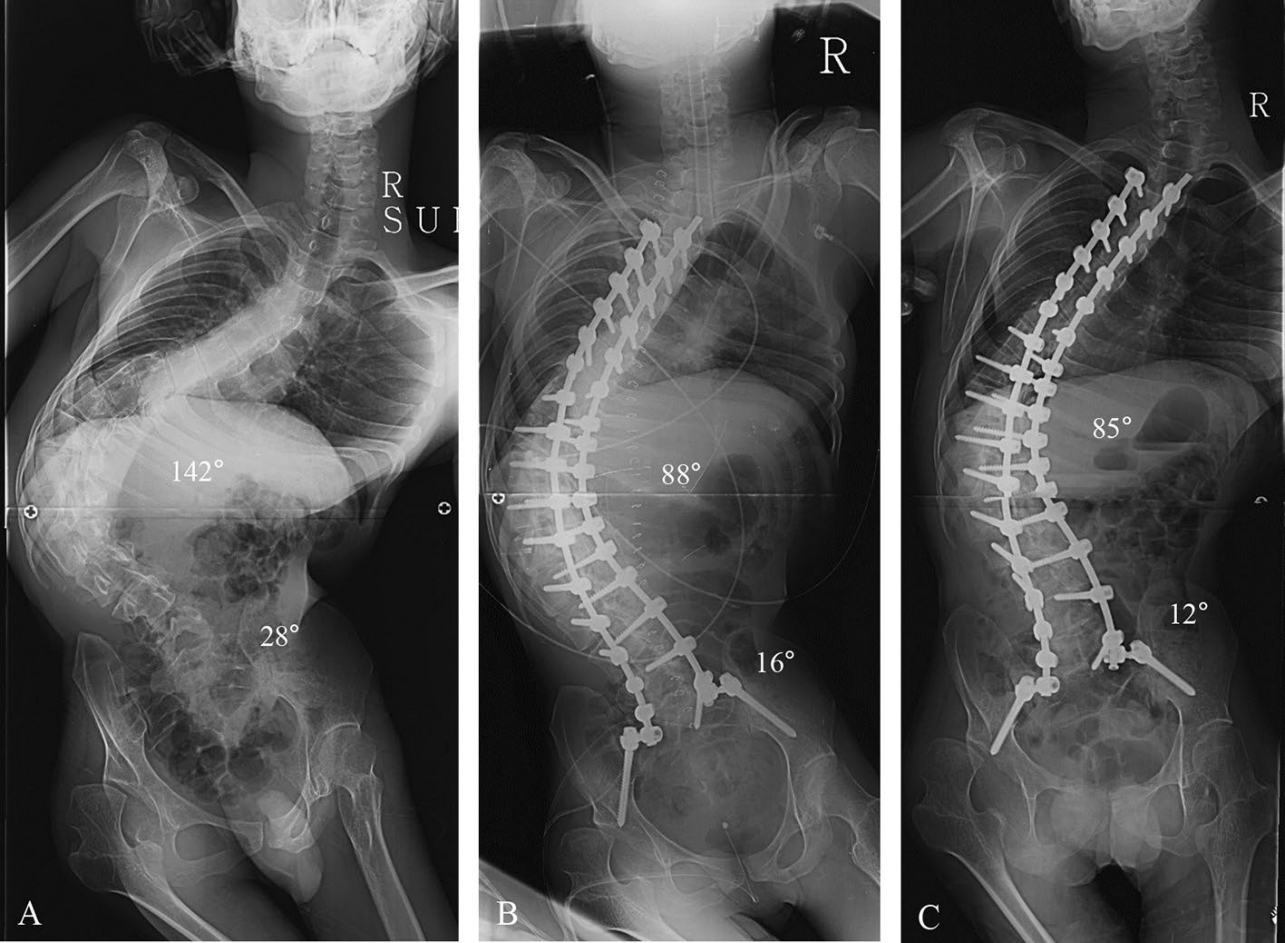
Deformity correction from T3 to spinopelvic fixation using iliac screw in patients with neuromuscular scoliosis (NMS). (A) A 27-year-old male patient who diagnosed with cerebral palsy (spastic quadriplegia) showed NMS. The whole-spine anterior–posterior image showed 142° of scoliosis deformity, and pelvic obliquity was 28° as measured by pelvic tilting angle. (B) The immediate postoperative radiograph showed 88° of the Cobb’s angle (61.4% of correction rate) and 16° of the pelvic tilting angle (42.9% of correction rate from the spinopelvic fixation). (C) The 2-year follow-up Cobb angle was 85° and pelvic obliquity was 12°.
